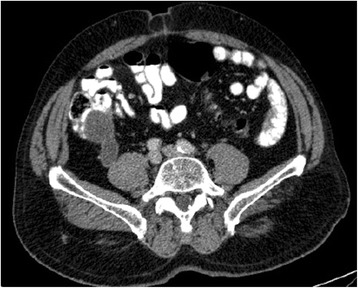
Axial computerized tomography view of a burst cecal appendix (up to 3.5 cm), with thin and regular walls, and no signs of densification of adjacent adipose tissue. This corresponds to the cystic formation already described in the ultrasound, compatible with mucocele of undetermined etiology. Appendectomy revealed well-differentiated mucinous adenocarcinoma, with invasion into of the muscularis mucosae
